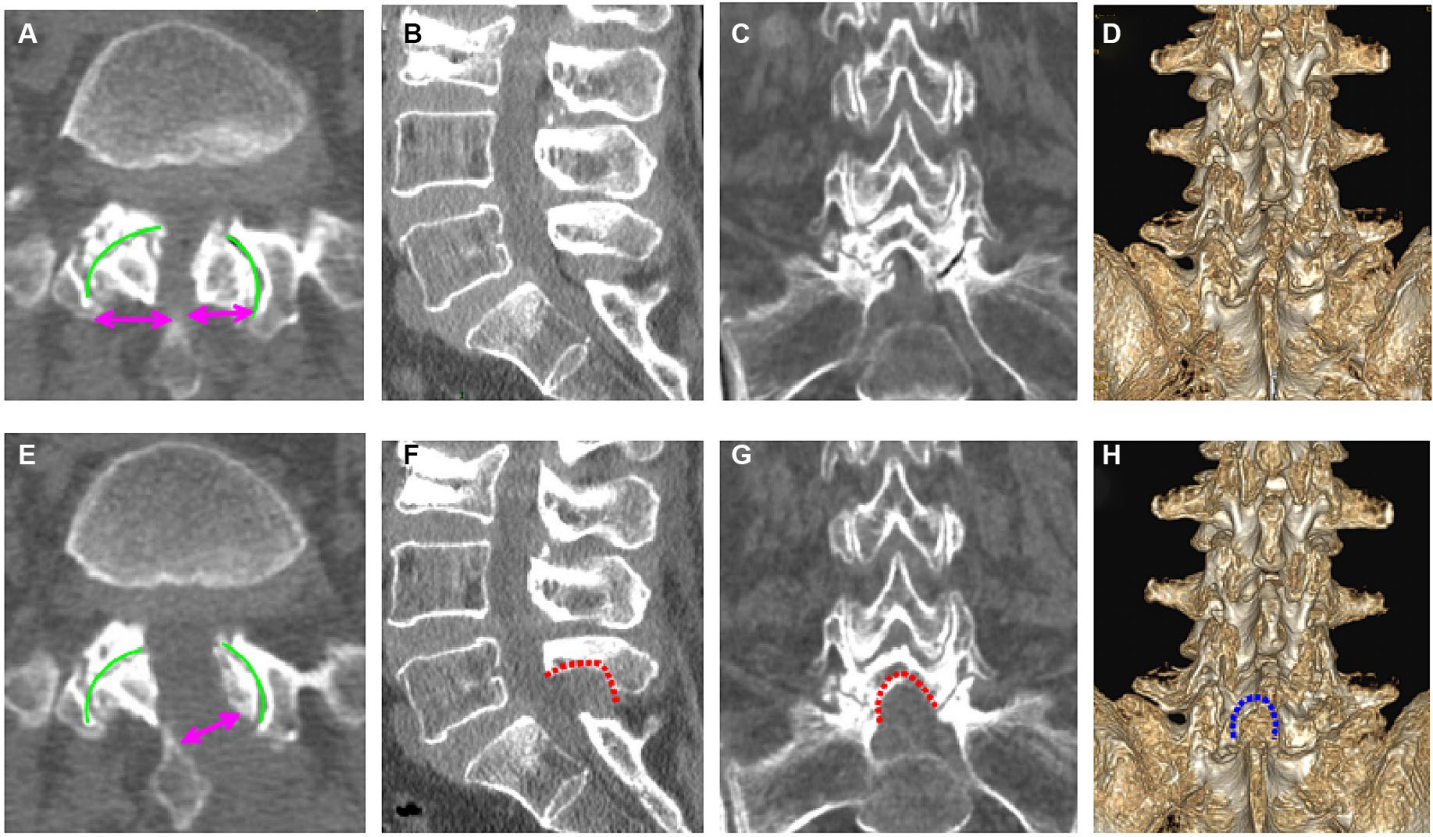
Pre- (A–D) and postoperative (E–H) computed tomography (CT) images of the lumbar spine (a 69-year-old woman). (A) Preoperative axial CT image of L5/S1; (B) preoperative sagittal CT image of L5/S1; (C) preoperative coronal CT image of L5/S1; (D) preoperative three-dimensional CT image of L5/S1; (E) postoperative axial CT image of L5/S1; (F) postoperative sagittal CT image of L5/S1; (G) postoperative coronal CT image of L5/S1; (H) three-dimensional CT image of L5/S1.
